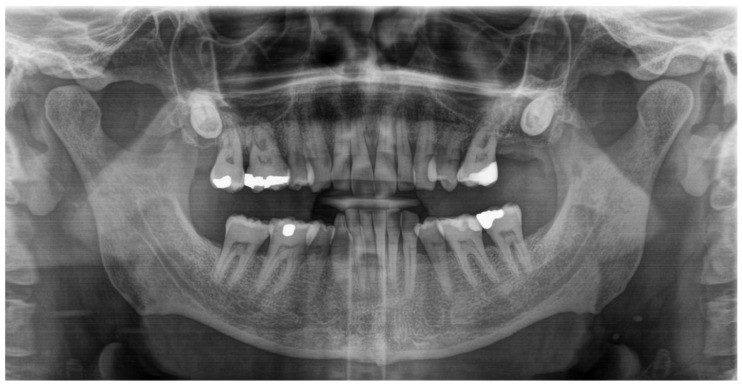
An orthopantomogram (OPG) was conducted as part of the X-ray-based diagnostic workup to evaluate the potential involvement of osseous structures within the jaws and surrounding anatomical regions that may have contributed to the lesion’s clinical presentation. The OPG revealed the absence of any underlying osseous pathologies or involvement of the mandible or maxilla, and did not reveal any pathological dental findings.
